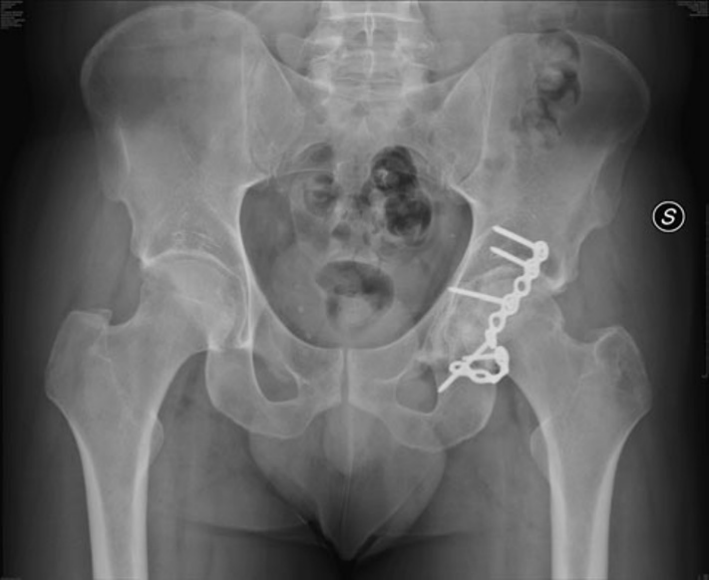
X-ray after 3 years showing a good clinical result
